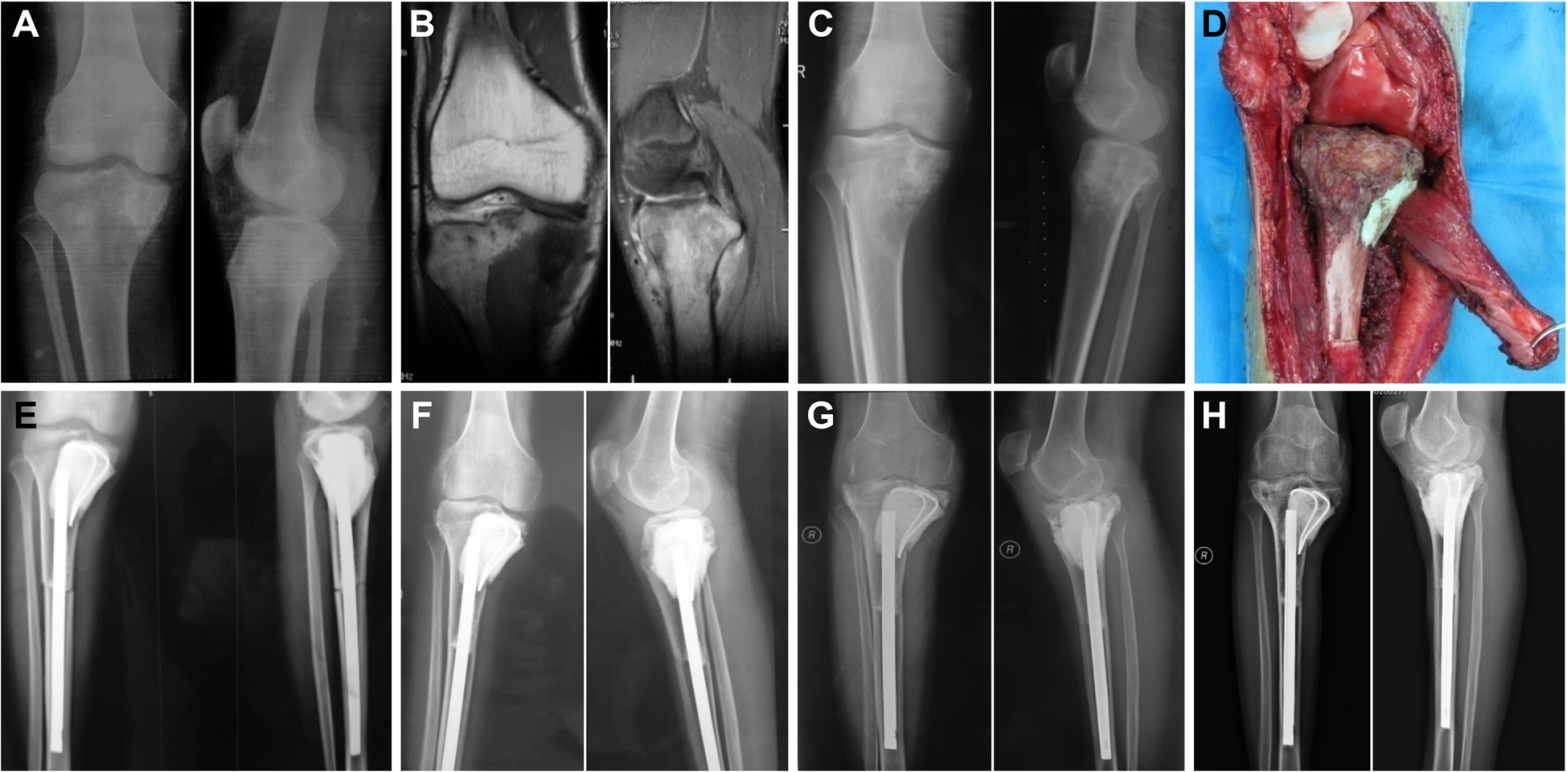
A Prechemotherapy X-ray examination showing osteolytic bone destruction in the right proximal tibia of an 18-year-old male patient who underwent joint preservation alcohol-inactivated autograft replantation for osteosarcoma. B Prechemotherapy coronal and sagittal MRI scans show a mix of high and low signals inside the medullary cavity of the tibia and surrounding soft tissue. C Two months after chemotherapy, the X-ray examination revealed that osteolytic bone destruction had markedly decreased. D The photo taken during surgery showed that the alcohol-inactivated autograft segment was filled with bone cement in the bone defect of the medial tibial plateau, replanted for reconstruction and firmly fixed by intramedullary nail fixation. E Radiographs taken 1 week after surgery showed solid internal fixation and joint preservation of the knee. F At 1 year after surgery, the X-ray examination revealed bone healing, but articular space narrowing was observed. G X-ray taken 3 years after limb salvage surgery. H At the 7-year follow-up, the X-ray examination of the right knee showed the condition of the alcohol-inactivated autograft segment. The subchondral bone was partially resorbed and fractured, narrowing of the space was observed, and arthrosis was indicated
